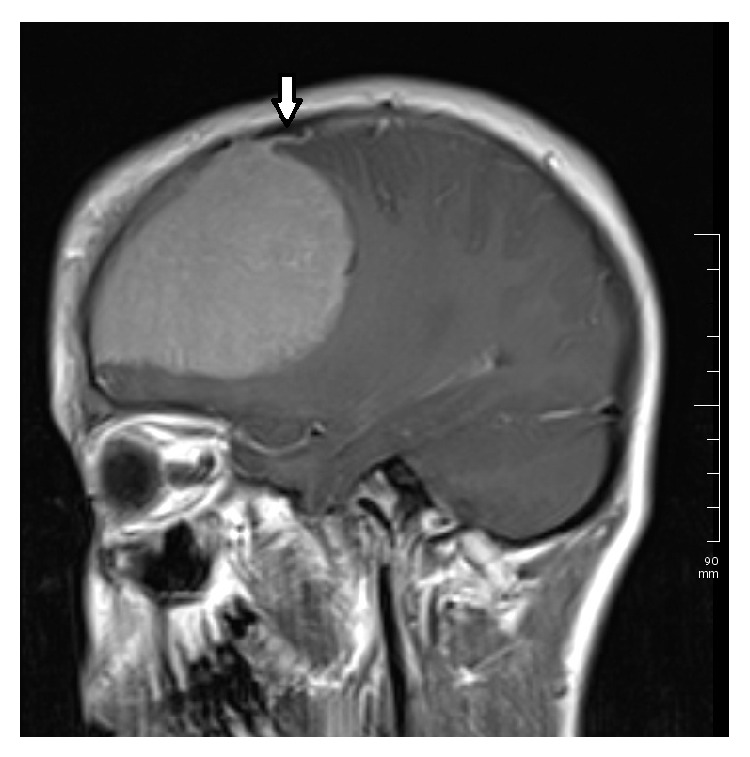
Magnetic resonance imaging. Sagittal T1-weighted MRI scan shows dural tail sign.
